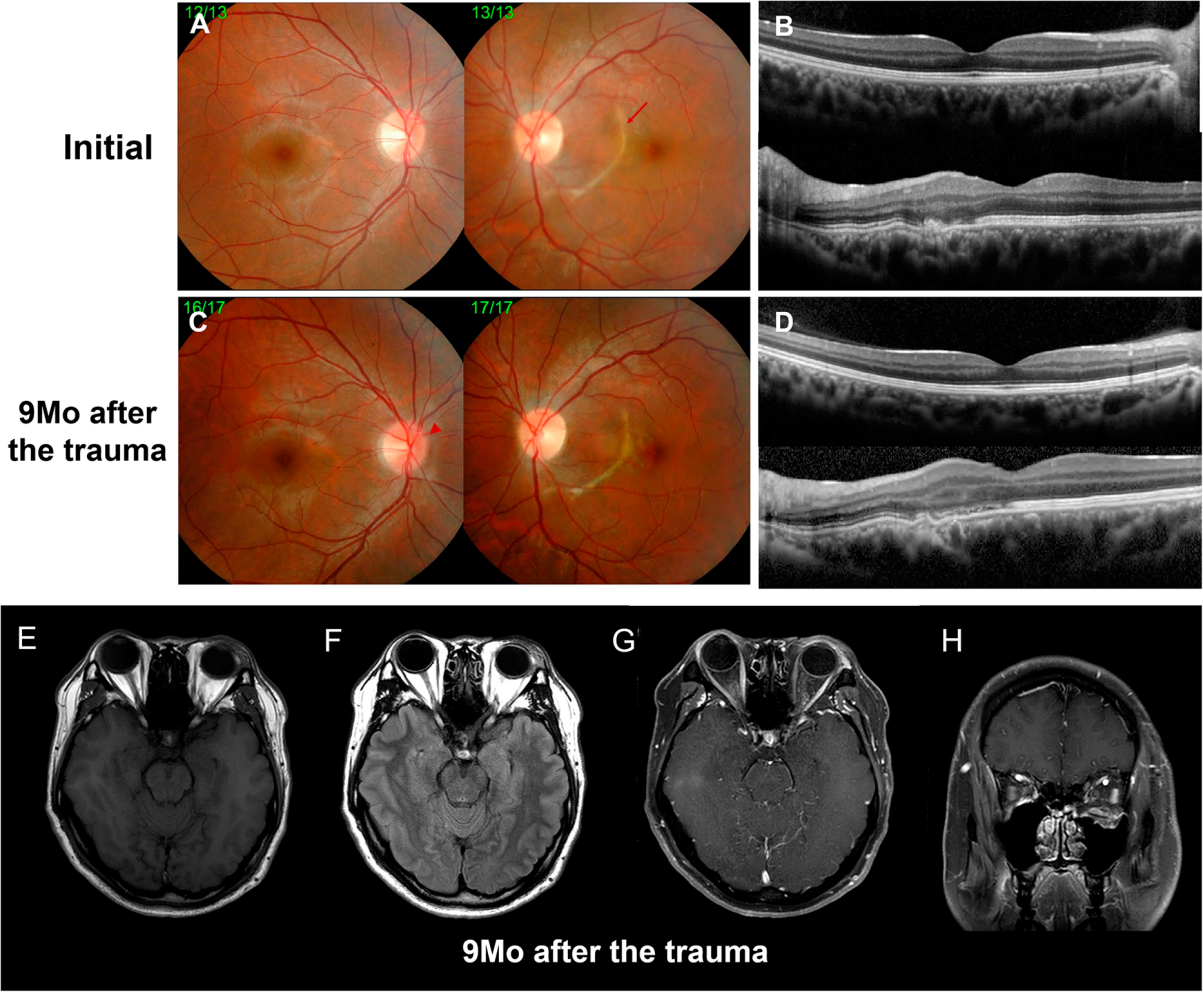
Comparison of fundus findings of the patient at initial visit and 9 months post-trauma, and a brain magnetic resonance imaging (MRI) with contrast enhancement that was performed after 9 months of the trauma. Initially, fundus photography (a, arrow) and optical coherence tomography (OCT) scan (b) revealed choroidal rupture in the left eye. However, the patient underwent central visual loss in the right eye 9 months post-trauma. No marked changes on the fundus (c) and OCT scan (d) are shown with the exception of the presence of a slightly hyperemic optic nerve head (arrowhead). No notable abnormal findings are shown in T1WI (e), T2WI (f), and contrast-enhanced MRI images (e–h)
